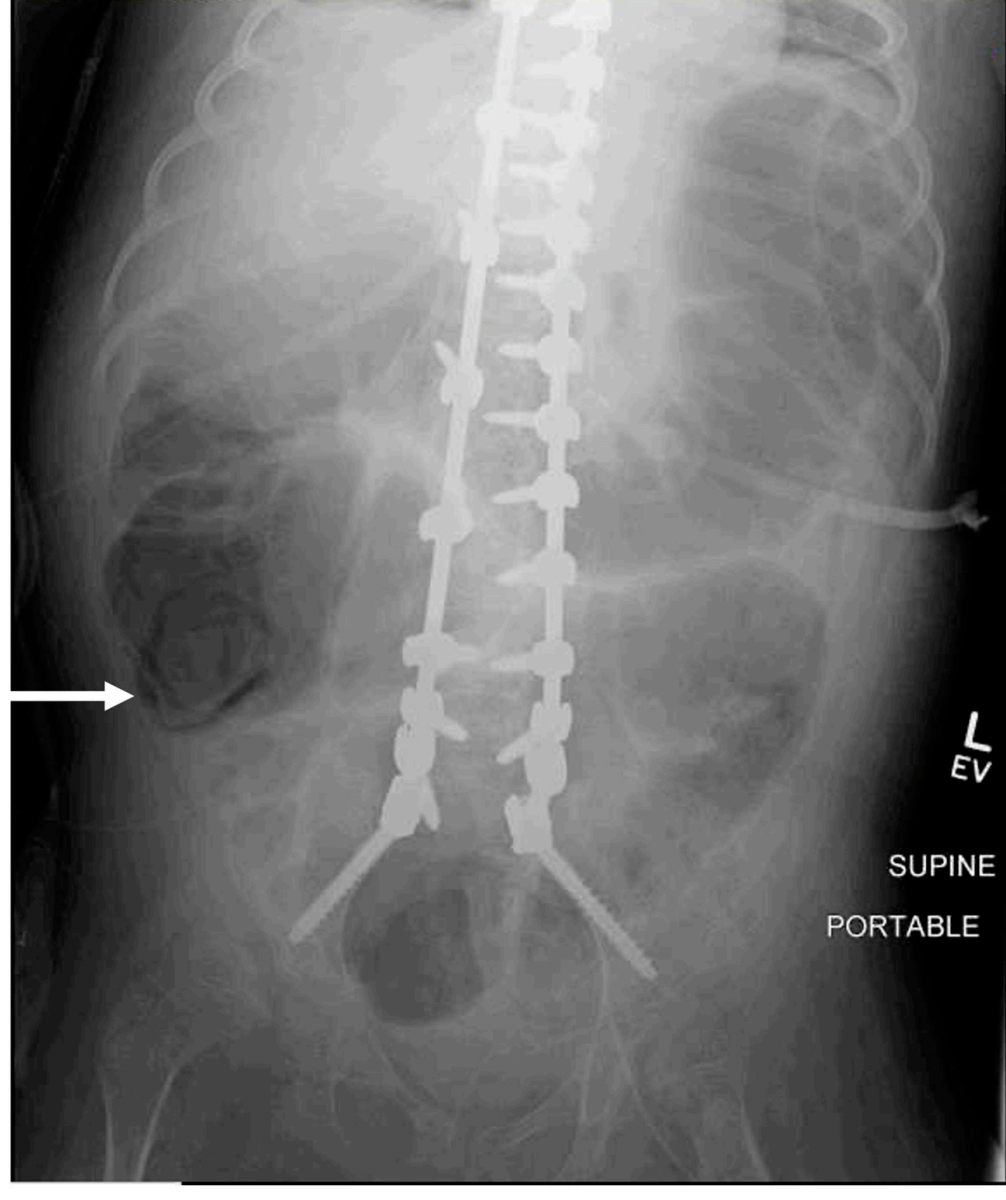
A supine AP abdominal radiograph of a nine-year-old male who underwent PSF for neuromuscular scoliosis was performed for worsening abdominal distension on postoperative day #2. The white arrow demonstrates the area concerning for possible pneumatosis coli, or free air within the wall of the colon.
